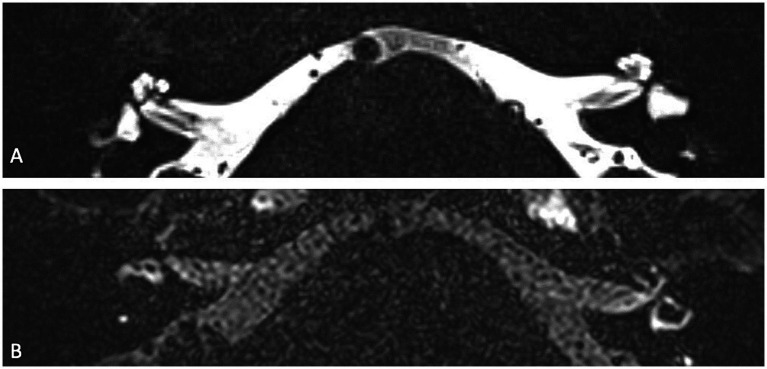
Axial 3D T2w TSE (A) and axial 4 h delayed post-Gd 3D Space FLAIR (B) showing a left-sided cystic dilatation of the saccule and utricle, that are fused on a congenital basis. On the delayed FLAIR image, there is a grade 2 vestibular hydrops (confluence of the saccule and utricle surrounded by a thin perilymphatic enhancement rim).
